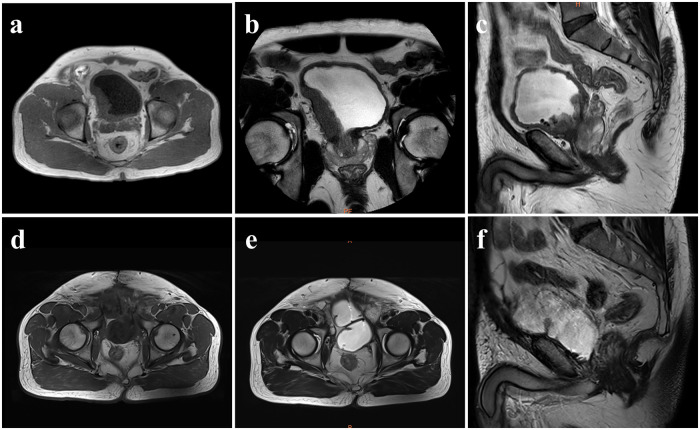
(a–c) Preoperative MRI image; (d–f) postoperative MRI image.
